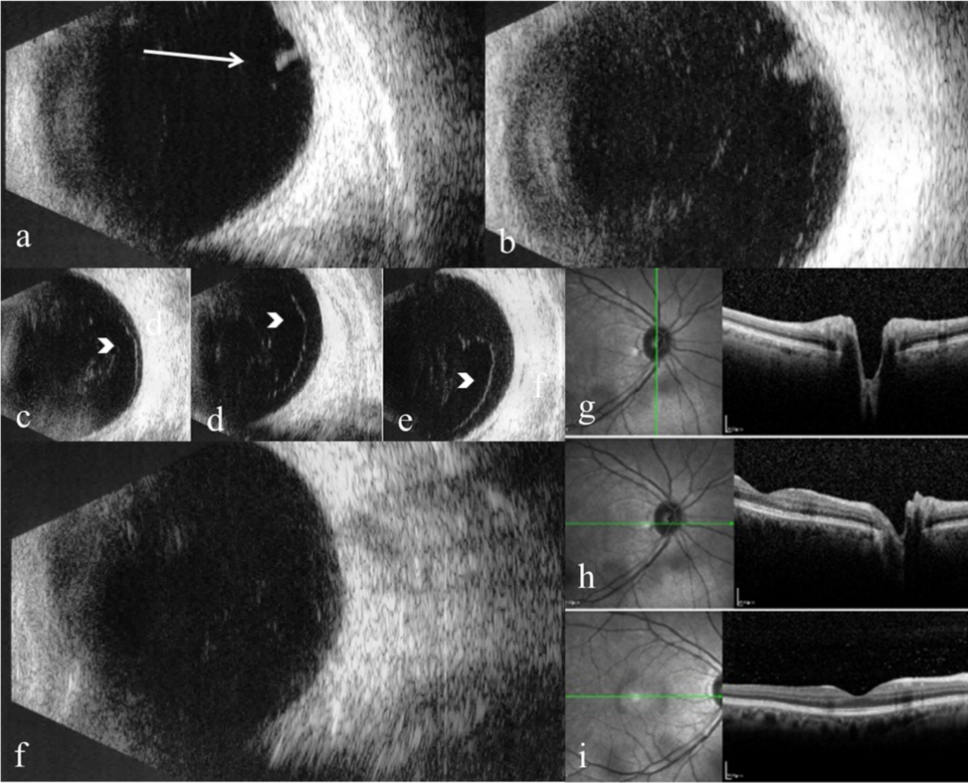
B-scan ultrasonography and optical coherence tomography showing extent of vitreous detachment. B-scan ultrasonography image showing retinal tear (arrow) (a); retinal tear with vitreo-retinal traction (b); anterior vitreous detachment (arrowhead) (c, d, e); posterior vitreous attachment (f); and optical coherence tomography images showing vitreous attached at the macula and optic nerve (g, h, i)
